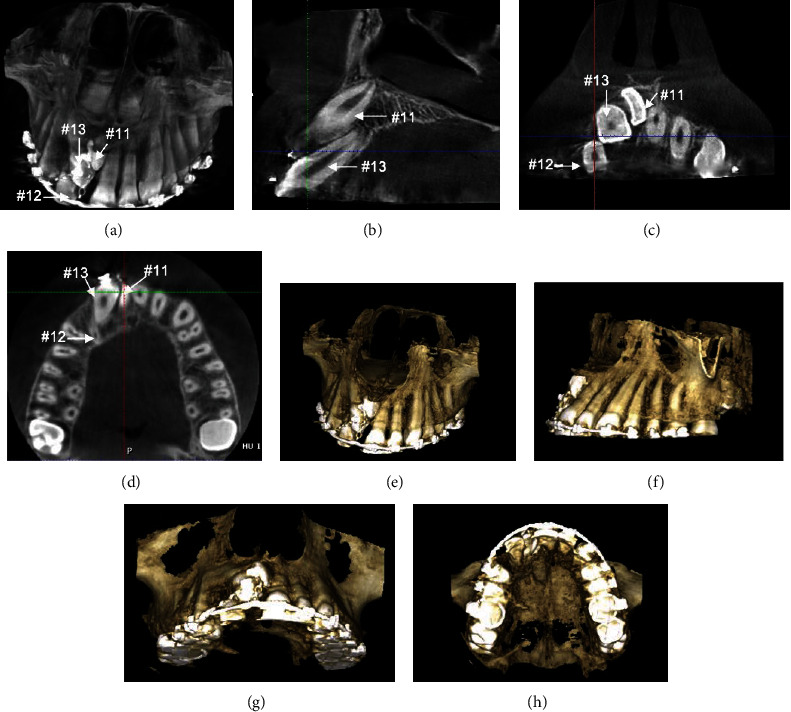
CBCT images of the maxillary anterior region immediately after surgical exposure and attachment bonding: (a) Frontal image. (b) Sagittal image. (c) Coronal image. (d) Axial image. (e–h) Three-dimensional reconstructed images.
